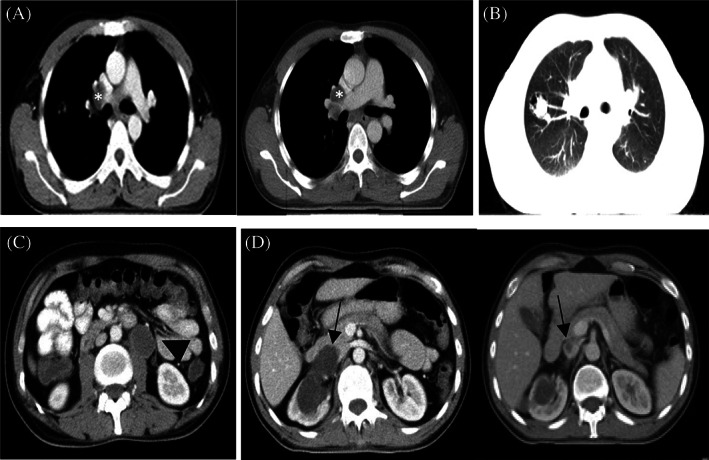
CT scan in March 2021. (A) Decreased size of lung mass, hilar, and mediastinal lymphadenopathies (asterisk). (B) 37 × 25 mm mass in upper lobe of right lung (parenchymal view). (C) A 34 × 27 mm paraaortic mass (arrow head). (D) A 77 × 33 mm cystic lesion with septa in middle pole of right kidney with extension to renal vein. The arrow shows IVC with a hypodense lesion in the center, which is actually along the tumoral thrombus of the renal vein.
